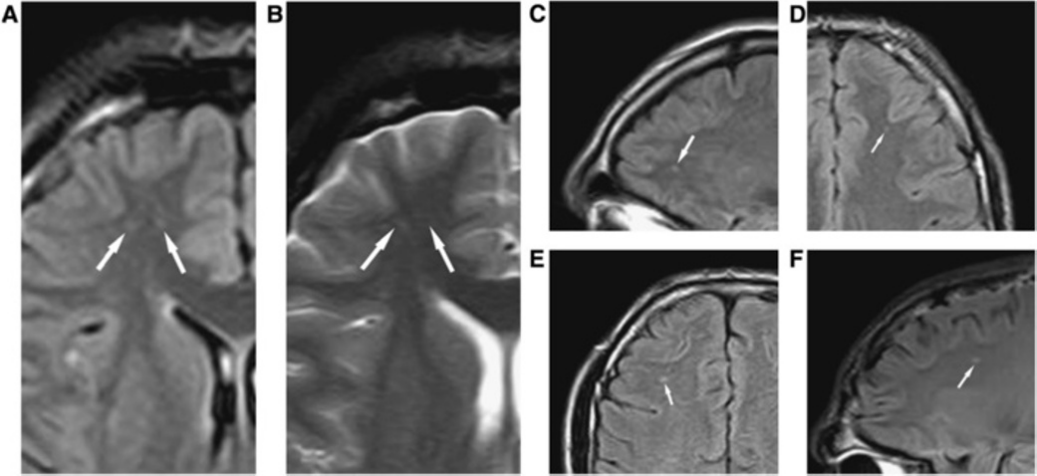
Diffuse axonal injury on MRI. (Panels A–F) Typical diffuse axonal injury, indicated by arrow. DAI was defined as focal areas of abnormal increased signal intensity on FLAIR and T2-­weighted sequences, measuring up to 5 mm in maximum diameter, and located at the gray matter = white matter interface or within or adjacent to the corpus callosum. In the Orrison *et al.* sample, 29% had DAI. Reproduced Ïrom Orrison *et al.*[107] with permission.
